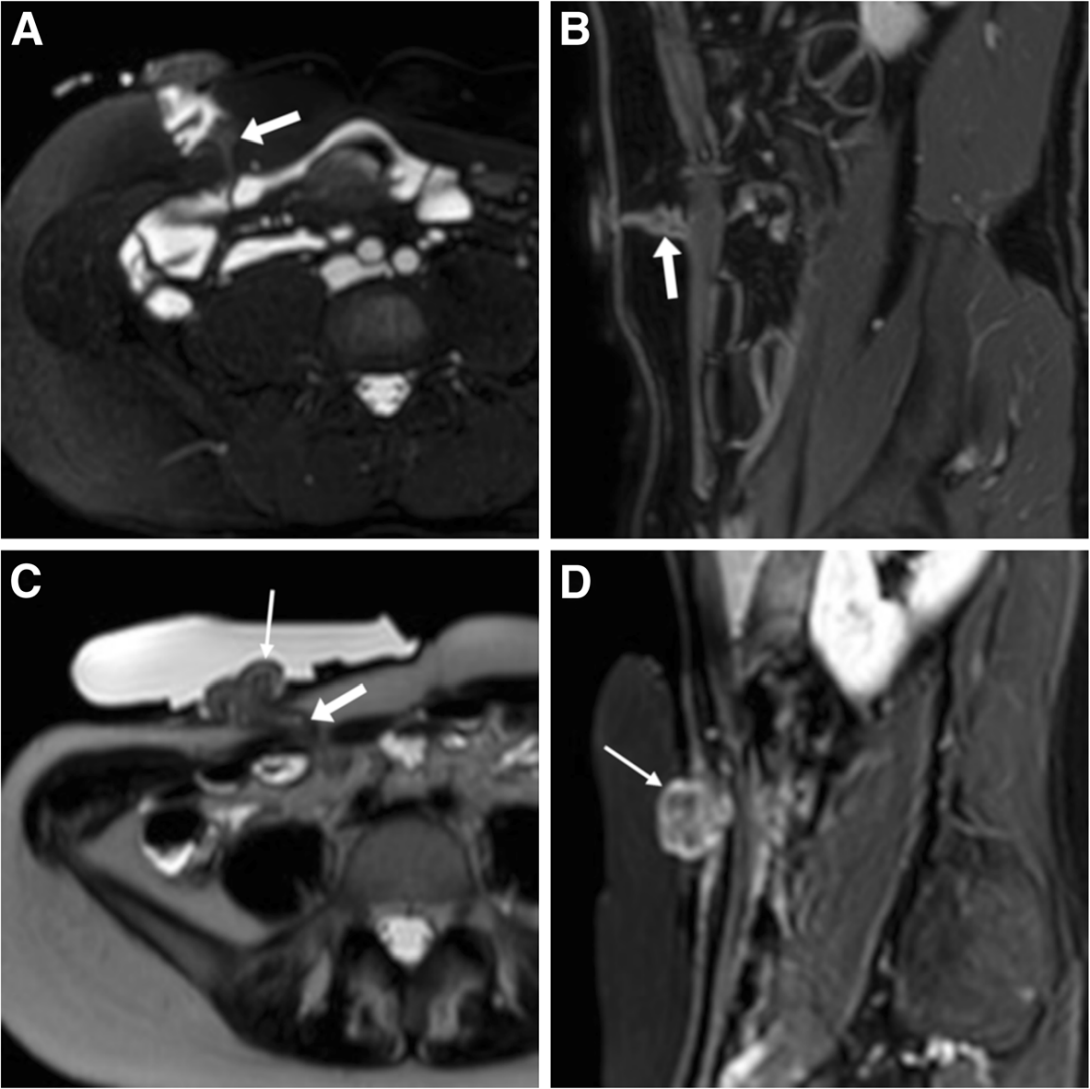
Normal appearance of ileostomies at MR-enterography in two patients operated for Crohn’s disease. a, b Axial fat-suppressed T2-weighted (a) and sagittal post-gadolinium fat-suppressed T1-weighted (b) images showing collapsed stomal tract (arrow) traversing the abdominal wall, with normal mural thickness and contrast enhancement comparable to that of other small bowel loops. c, d Axial T2- (c) and post-gadolinium fat-suppressed T1-weighted (d) images showing collapsed stomal tract (arrow), mild protrusion at external orifice (thin arrows), and abundant fluid flowing within stoma bag consistent with stomal patency
